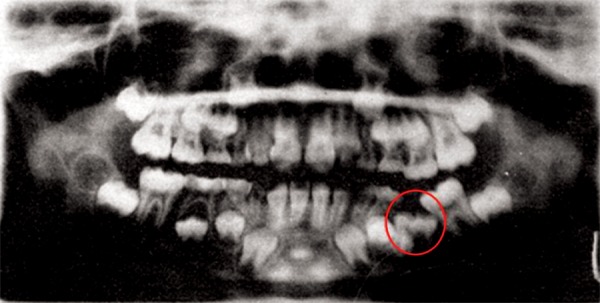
Panoramic radiograph showing a submerged deciduous mandibular left second molar
